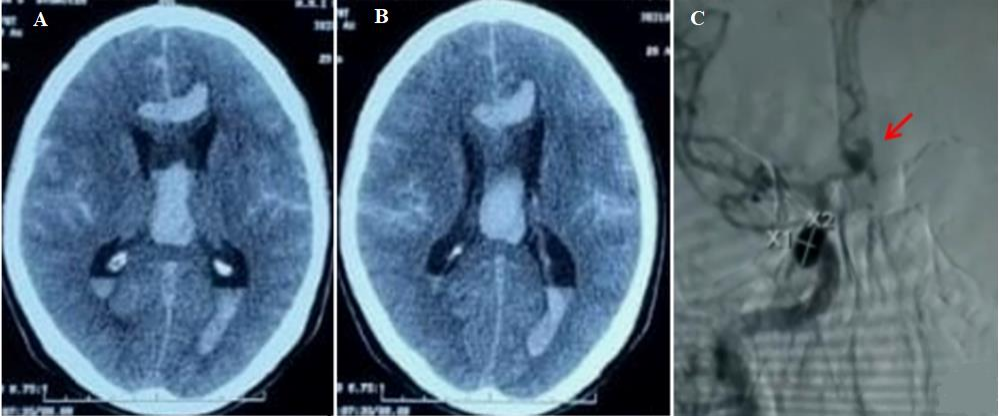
A,B) unenhanced cerebral computed tomography showing a hyperdensity in the subarachnoid space with corpus callosum hematoma, septum pellucidum hematoma and intraventricular hematoma; C) cerebral angiography showing an anterior communicating artery aneurysm, red arrow
